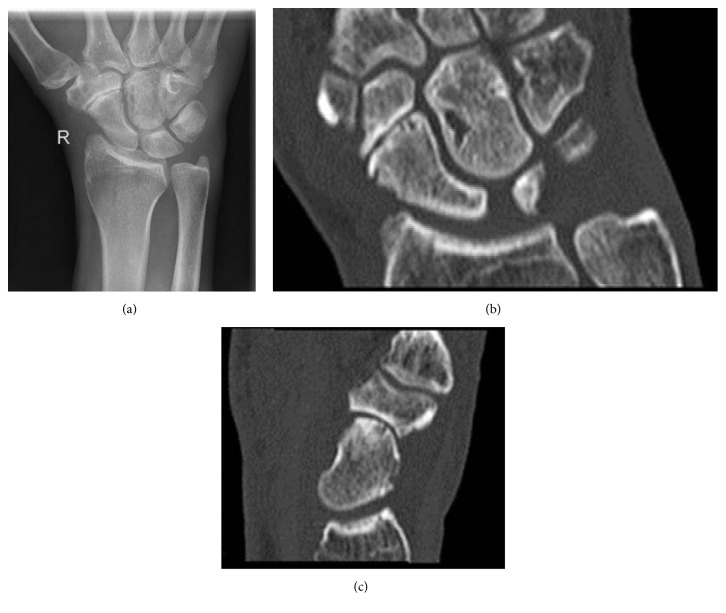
(a) X-ray of right wrist showing fracture of the scaphoid and preexistent osteoarthritis of the STT-joint. (b, c) Preoperative CT-scan demonstrating fracture of scaphoid classified as Herbert B2 but also clearly demonstrating degenerative changes of the carpal bones.
